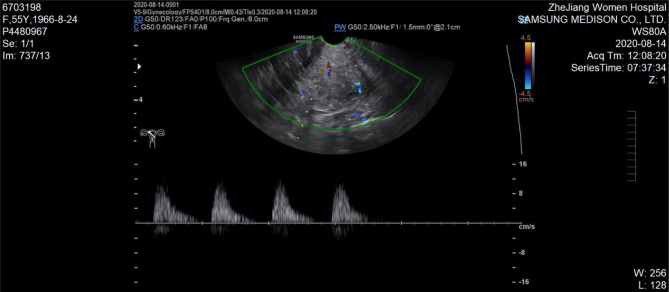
Ultrasonography showed echogenicity of thickened myometrium, suggesting adenomyosis.
